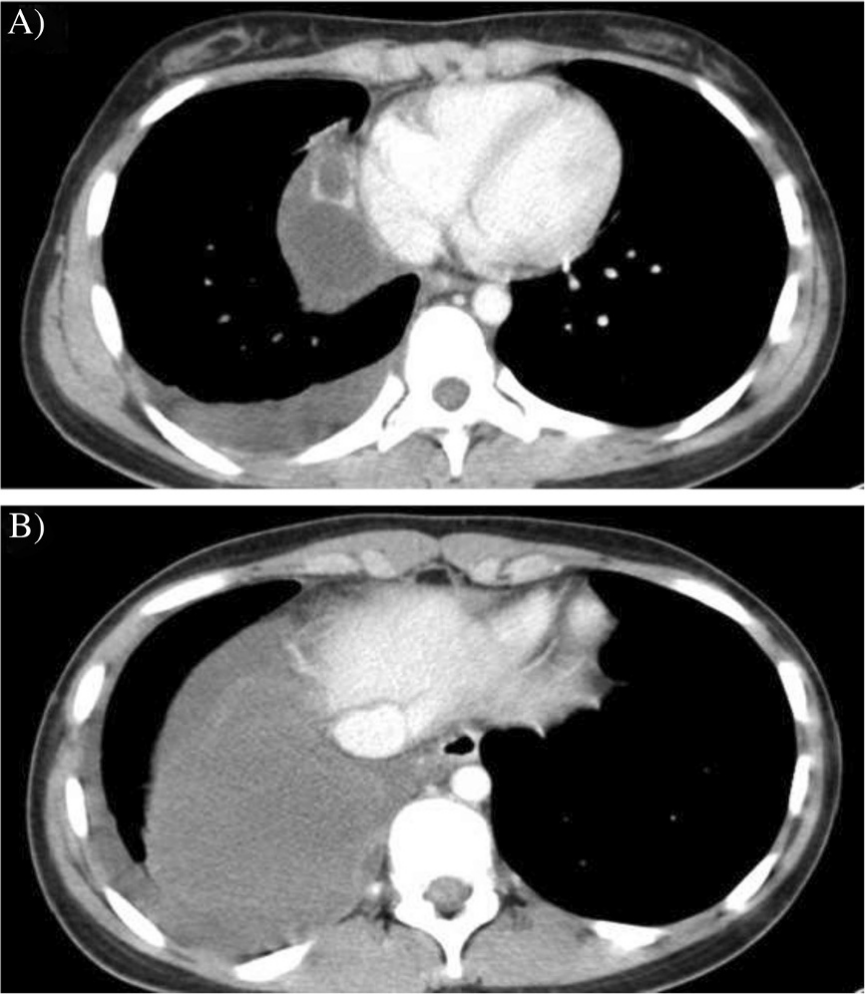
Computed tomography imaging. (A) Computed tomography revealed lobulated cystic masses with an enhanced tumor wall in the anterior mediastinam. (B) Computed tomography revealed a low density area equal to pleural effusion with an adjacent thin wall.
